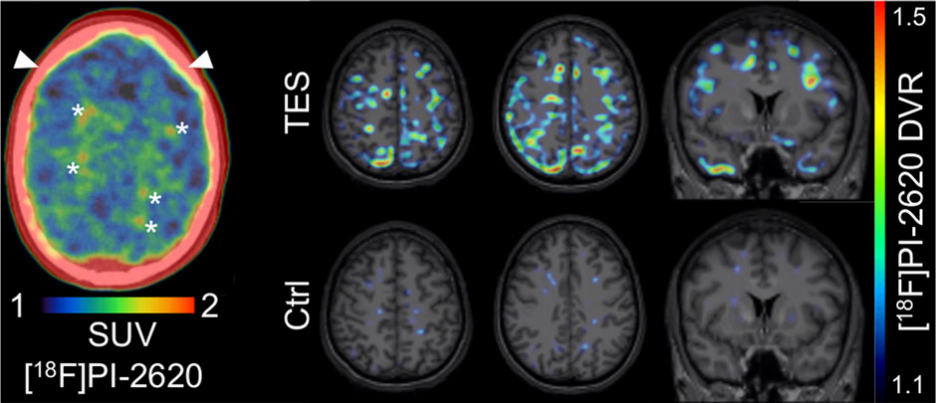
Tau-PET. Left, static tau-PET/CT image (20–40 min p.i.) as assessed in clinical routine, indicating focal lesions of increased tracer uptake at the frontal and parietal white/gray matter border (*) and elevated skull uptake (white arrows). Right, parametric semiquantitative tau-PET images derived from the full 60-min scan (DVR), upon an MRI template (masked extracerebral structures). Upper row shows the patient, lower row shows a tau-negative control (47 years, male). Semiquantitative comparison confirmed elevated focal tau-PET signal predominantly observed at the white/gray matter border in the patient. A dynamic 60-min PET emission was recorded upon bolus (10-sec) tracer injection using a Siemens Biograph 64 PET/CT scanner (Siemens Healthineers, Erlangen, Germany). A low-dose CT served for attenuation correction. Imaging data were reconstructed using an iterative OSEM3D algorithm and binned into 35 frames (12 × 5, 6 × 10s, 3 × 20s, 7 × 60s, 4 × 300s, 3 × 600s). Multi-linear reference tissue modeling 2 was performed using the cerebellum (excluding the dentate nucleus and superior layers) as a reference tissue. For simplified quantification, the 20- to 40-min SUV was calculated. Parametric data of the patient were visually compared to a cognitively unimpaired 47-year-old male after projection upon an MRI template. CT, computed tomography; DVR, distribution volume ratio; MRI, magnetic resonance imaging; PET, positron emission tomography; SUV, standard uptake value.
